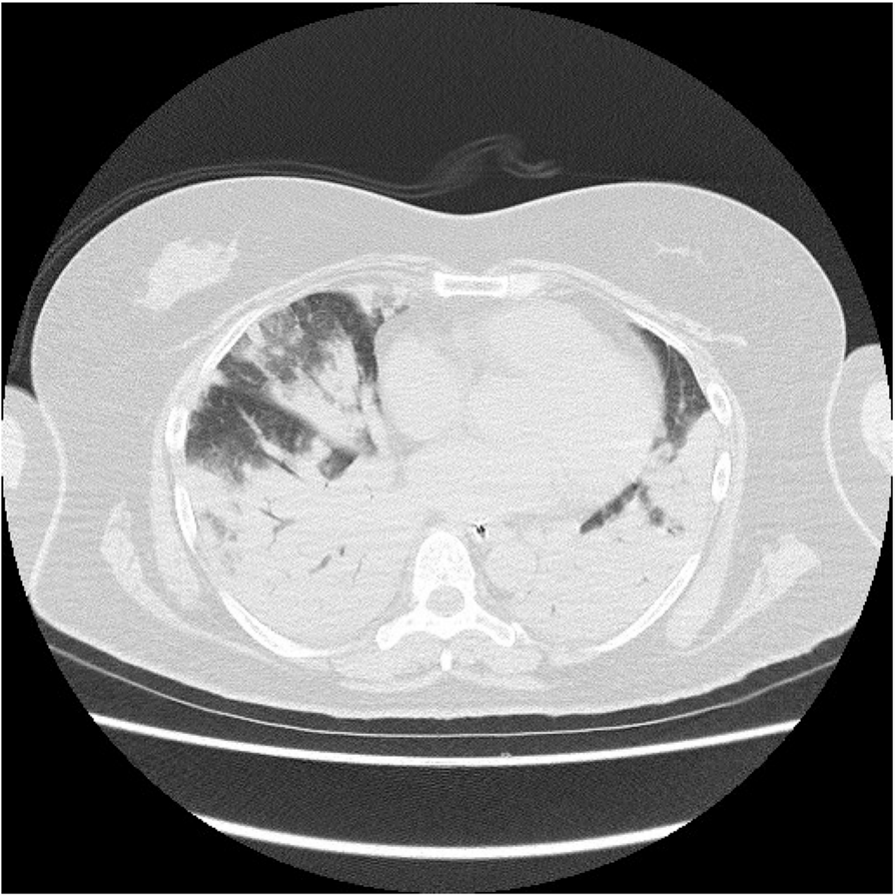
Chest computed tomography findings show infiltrating shadows that predominate in the bilateral lower lung fields
